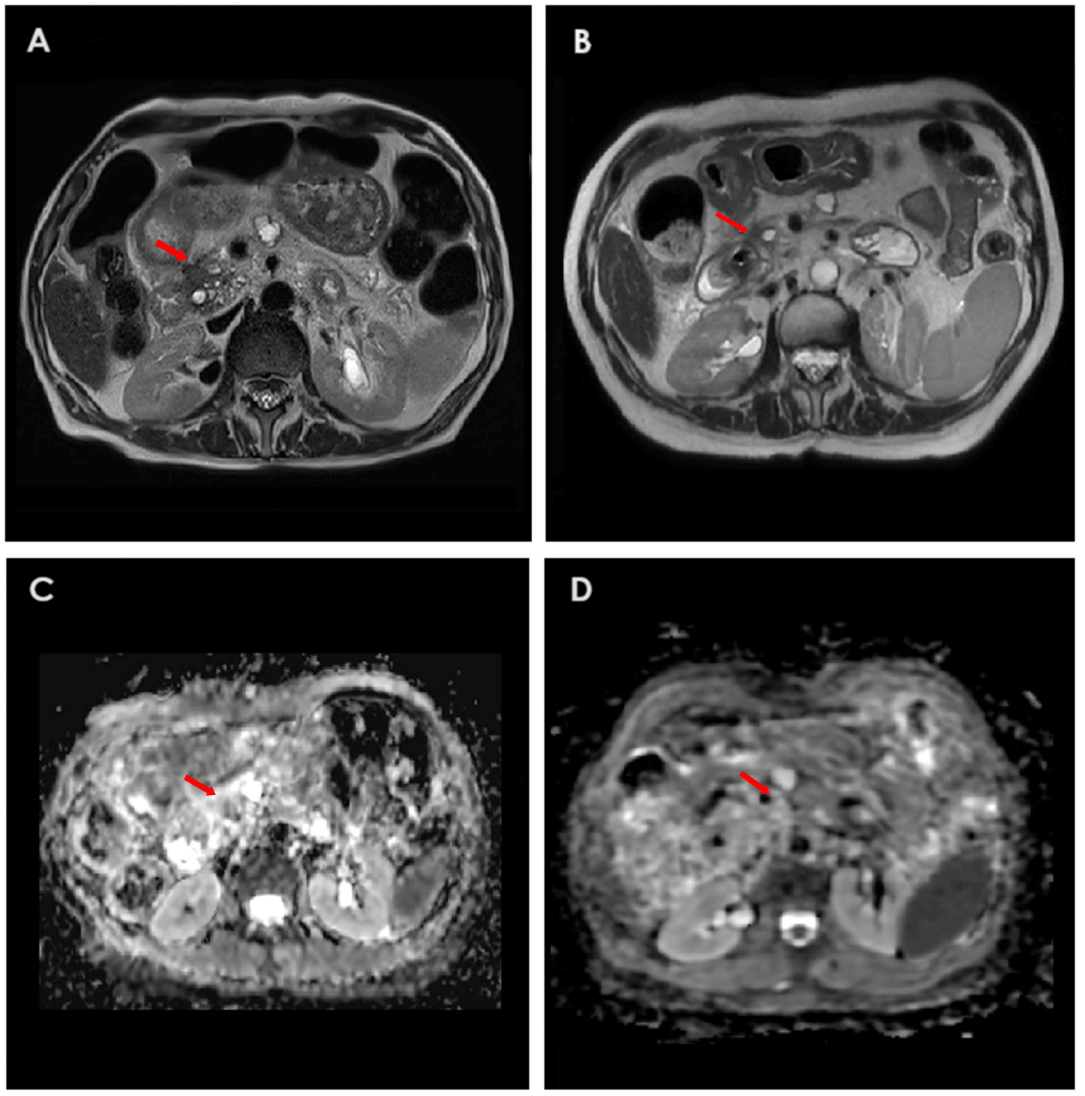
Abdominal CE-MRI images of the primary pancreatic tumor during systemic treatment and after pancreatic SBRT. (A) T2-weighted sequence on April 23, 2024, during chemotherapy, shows a 20 mm pancreatic head mass (red arrow) with ductal dilatation. (B) T2-weighted sequence on May 5, 2025, post-SBRT, demonstrates complete tumor resolution (red arrow) with moderate pancreatic atrophy. (C): ADC map on April 23, 2024, shows a pancreatic mass with diffusion restriction, indicating metabolic activity (viability). (D): ADC map on May 5, 2025, shows an absence of diffusion restriction in the lesion, consistent with non-viability of the tissue. CE-MRI: contrast-enhanced magnetic resonance imaging; SBRT: stereotactic body radiotherapy; ADC: apparent diffusion coefficient
